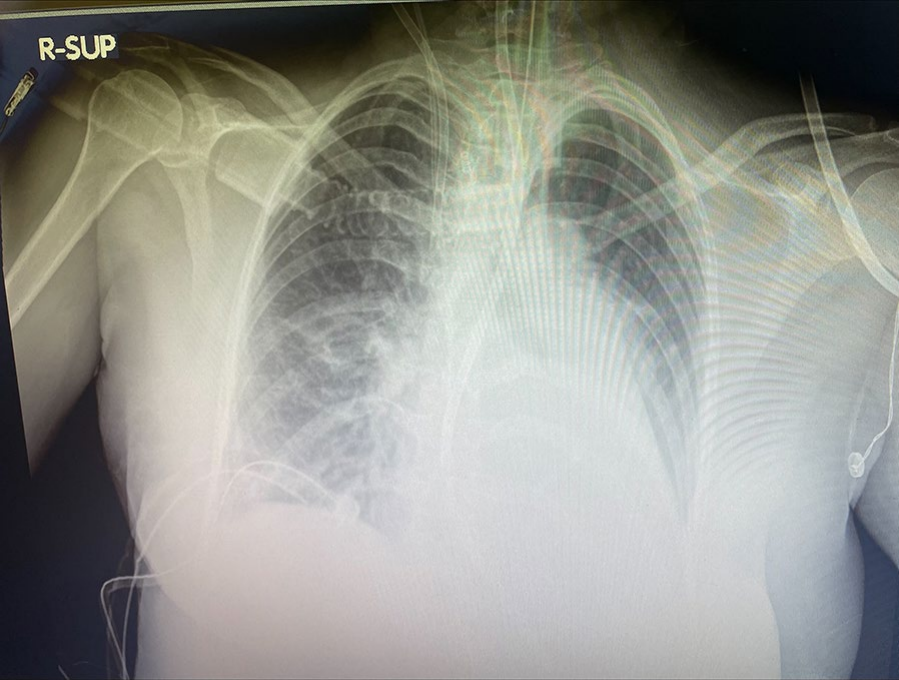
The chest X-ray after surgical procedure
